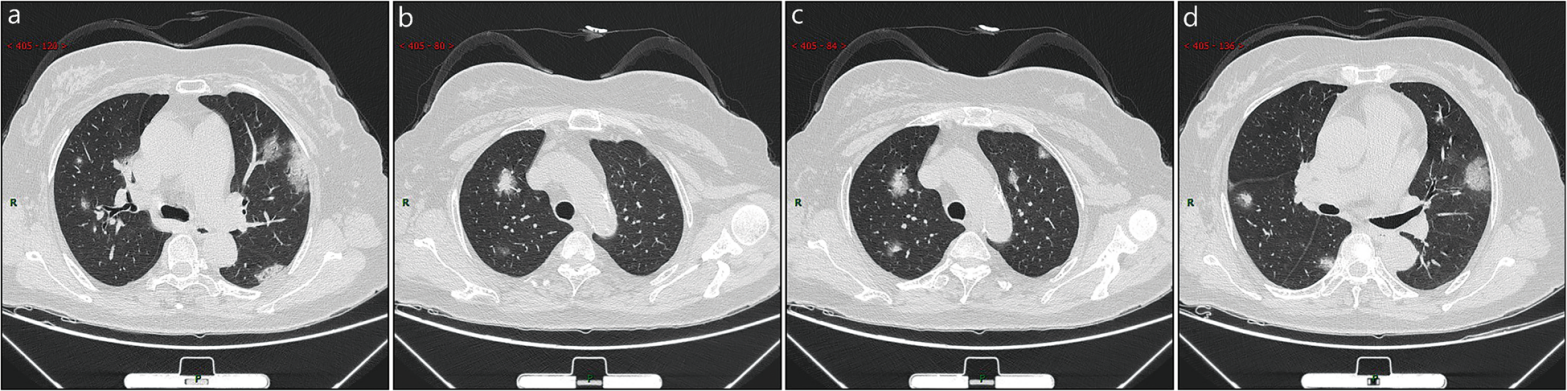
Atypical CT / X-ray imaging manifestation (case 2). A 56 years old female with fever for 3 days. Laboratory test: decreased total protein (54.0 g/L),decreased albumin (35.5 g/L),decreased globulin (18.5 g/L), normal white blood cells (4.87 × 10^9^/L), decreased lymphocytes percentage (10.1%), decreased lymphocytes (0.49 × 10^9^/ L), decreased eosinophil count (0 × 10^9^/L)), decreased eosinophil count percentage (0%). Imaging examination: a showed two consolidation nodulesat the center of the lateral segment of middle lobe of the right lung which was surrounded by ground-glass opacities; b showed patchy ground-glass opacity in the anterior segment of the right upper lung with patchy consolidation lesions in it; c showed patchy ground-glass opacities in both lungs with patchy consolidation lesions in it. d showed patchy consolidation in the ground-glass opacities in the middle lobe and dorsal segment of lower lobe of right lung
